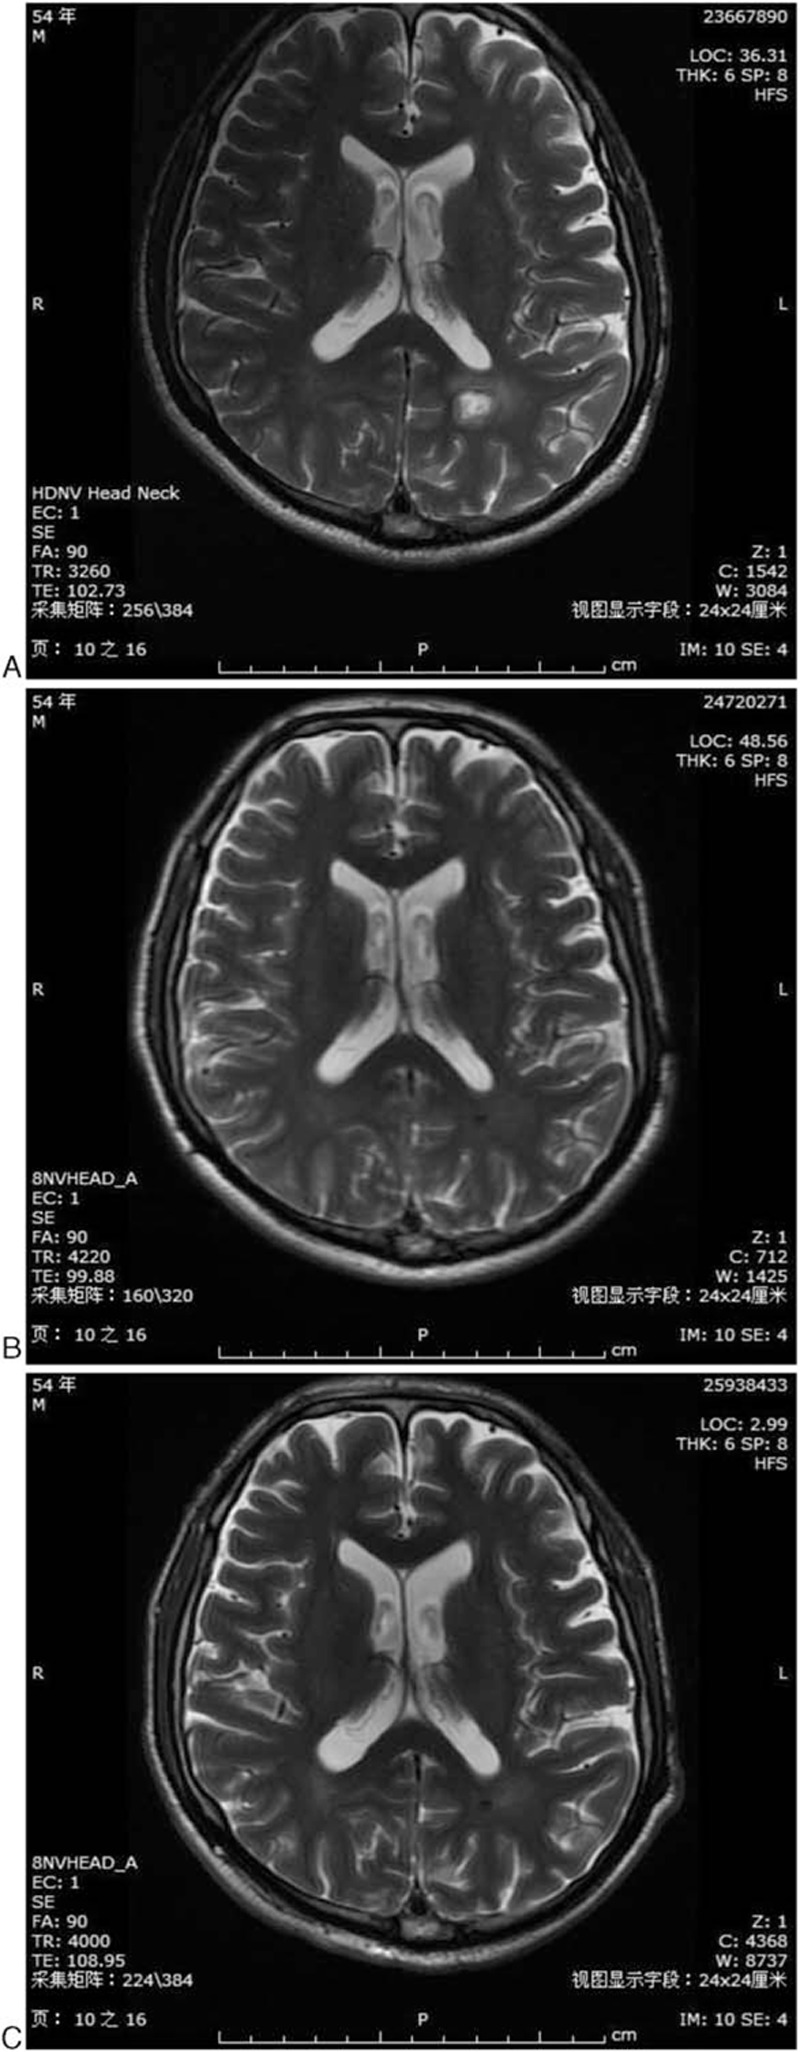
Image of brain magnetic resonance imaging. (A) Before chemotherapy (November 2014); (B) After chemotherapy of pemetrexed/cisplatin for 6 cycles (April 2015); (C) After radiotherapy combined with chemotherapy of pemetrexed for 3 cycles (September 2015).
